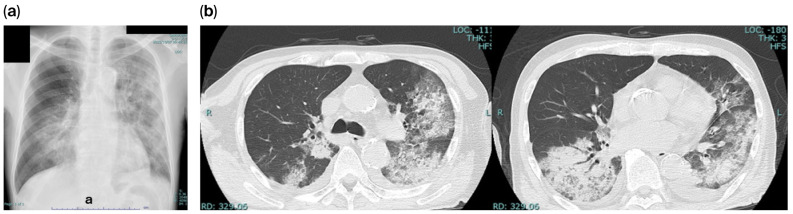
Chest X-ray and Computed tomography (CT) at the time of admission. (a) The X-ray images showed ground-glass opacities in both lungs, with infiltrates within them. (b) Chest CT images showed ground-glass opacity (GGO) with a crazy-paving pattern as the main feature in the upper lobes, accompanied by partial infiltrative shadows within them. In the lower lobes, ground-glass opacity was partially observed, but consolidation was predominant. Significant bilateral pleural effusions were seen.
